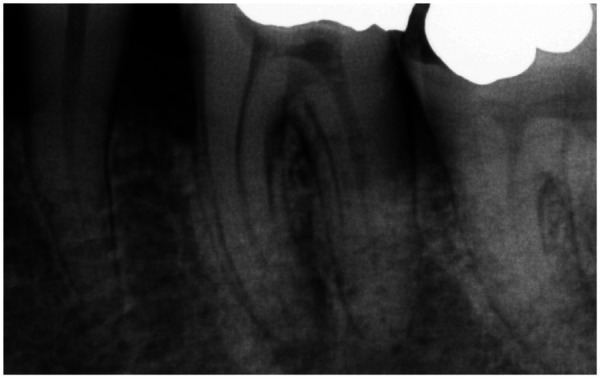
Initial intraoral radiograph of tooth 36.
